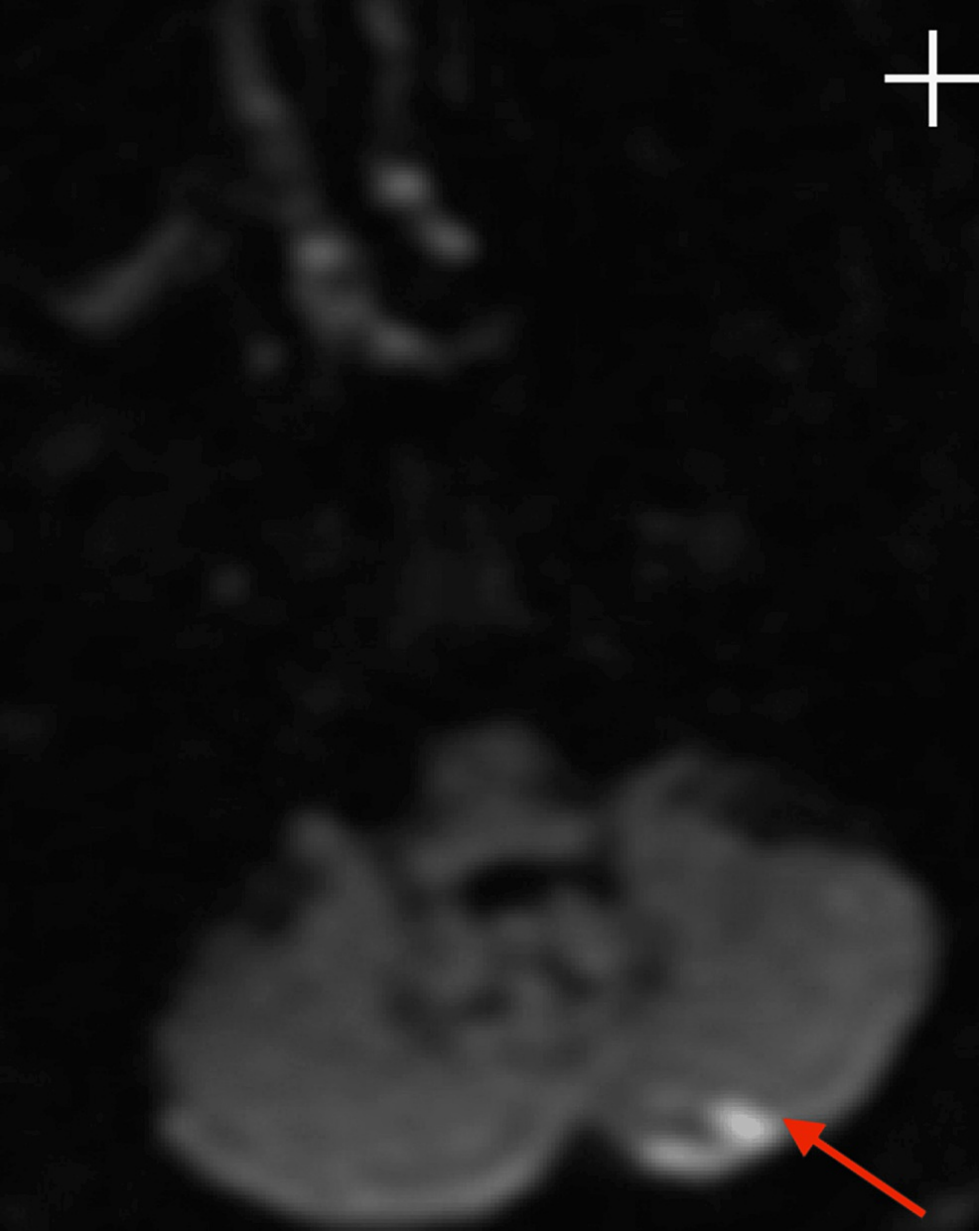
Brain MRI DWI Axial Acute infarct involving the medial left cerebellar hemisphere. DWI: Diffusion-weighted imaging
